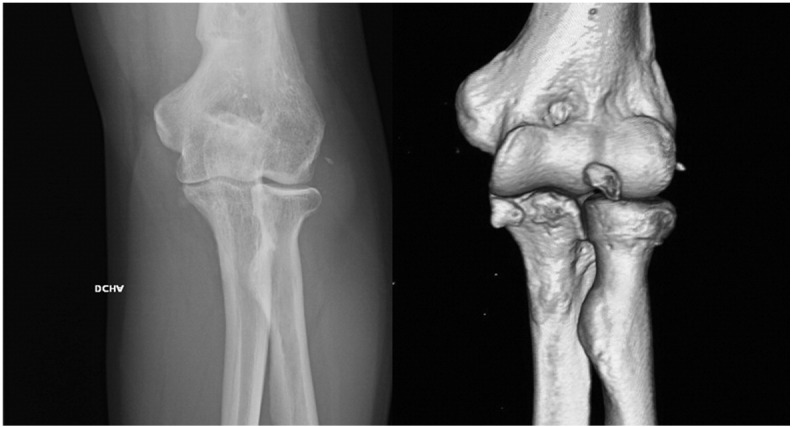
Six weeks radiographic examination of the right elbow shows medial joint space reduction so an anteromedial coronoid fracture was suspected and a CT scan was performed. A small anteromedial coronoid fracture is observed along with a fracture of the coronoid (O'Driscoll type II fracture). At this point in time, the reconstructive options are limited.
